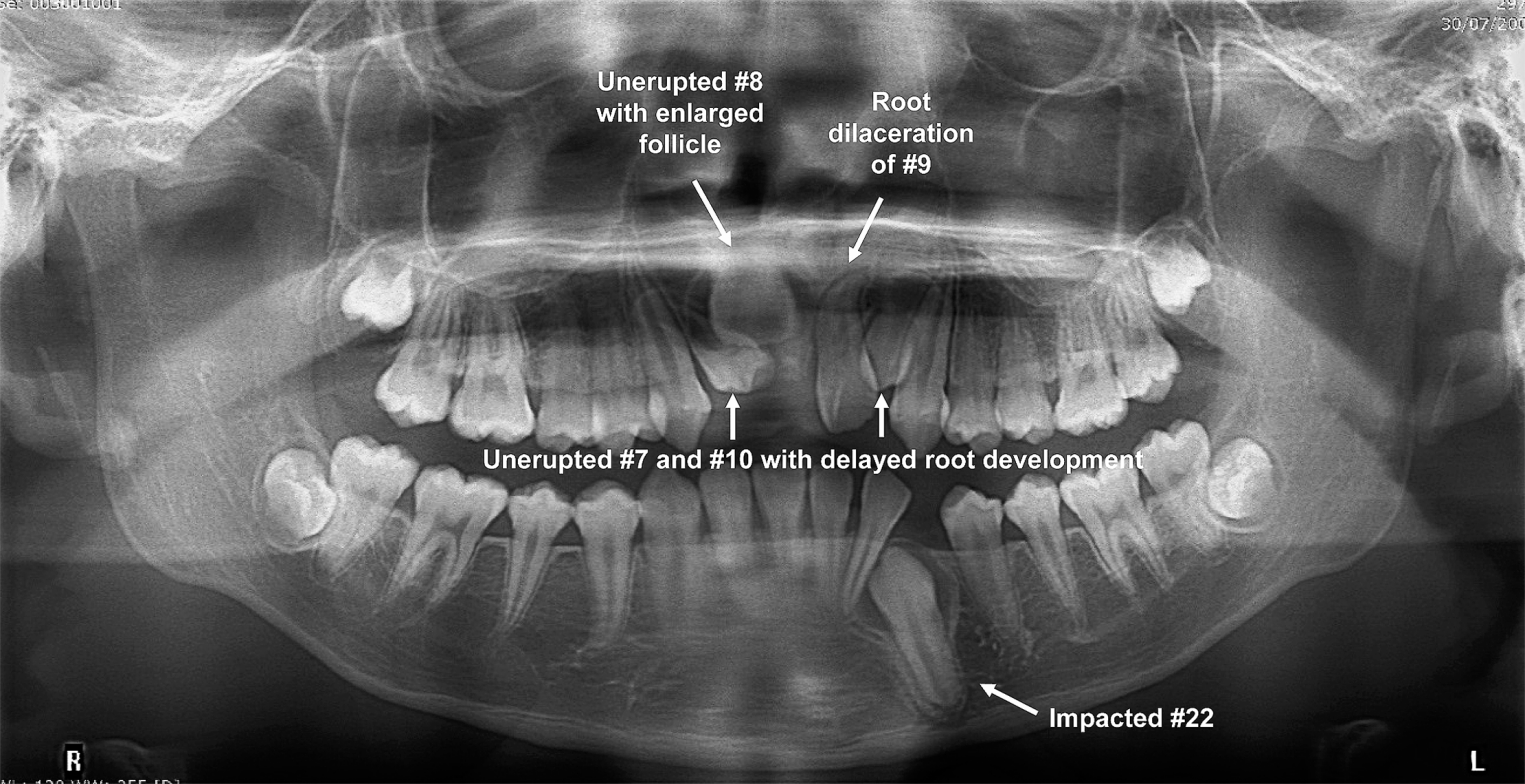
Panoramic radiograph of a 14-year-old girl (with no history of dental trauma) presented with root dilaceration of #9, unerupted #8 with enlarged follicle, unerupted #7 and #10 with delayed root development; as well as an impacted #22.
